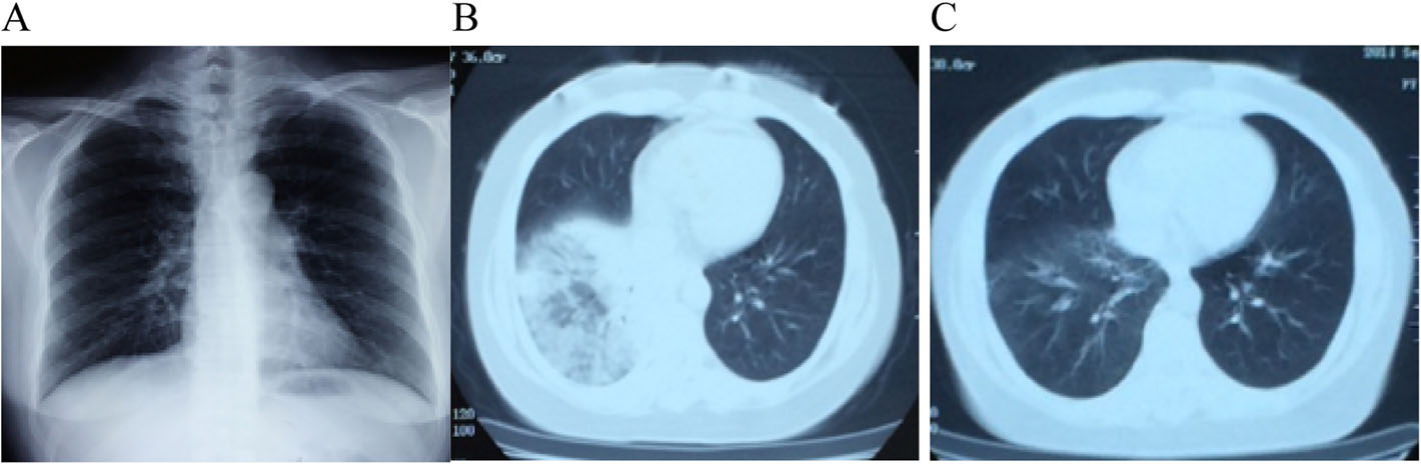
Chest images before or after the first BT session. a Chest X-ray was normal before the right lower lobe BT treatment. b Chest CT revealed infiltrative shadows in the right lower zone with air bronchogram sign after the right lower lobe BT procedure. c Chest CT showed the infiltrates in the right lower lobe had significantly resolved after one-week of antibacterial treatment
